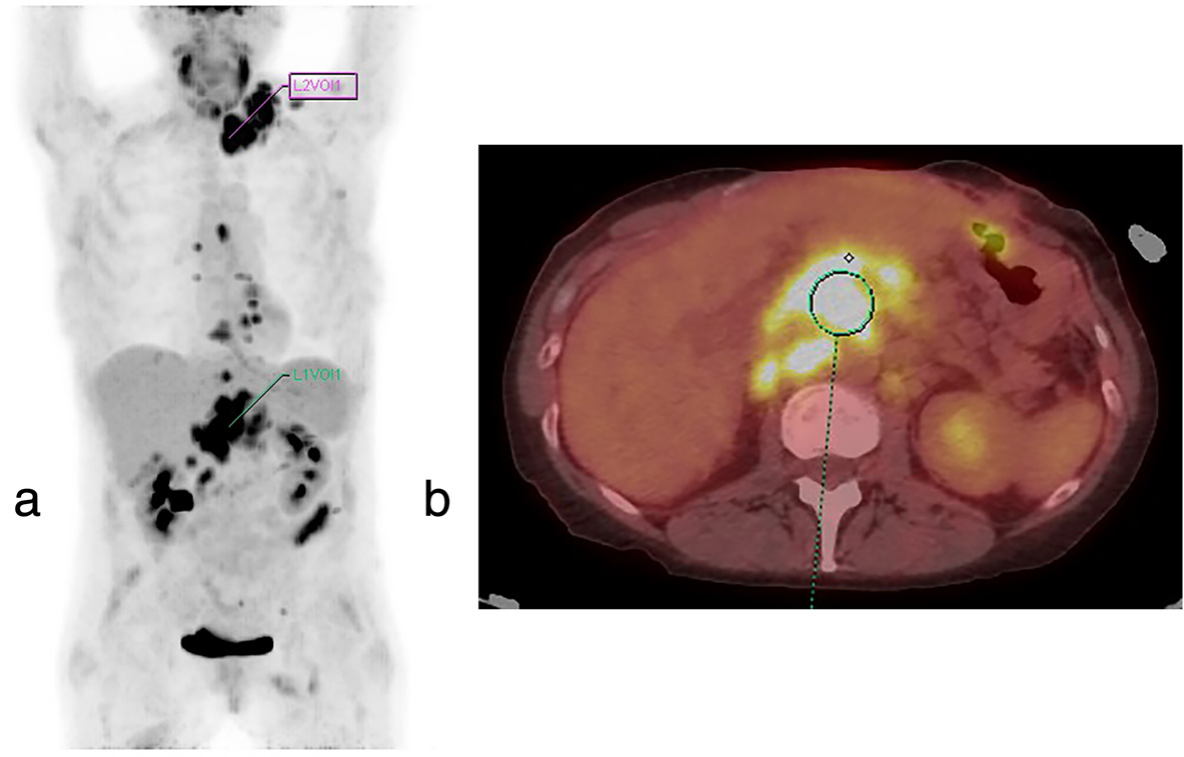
PET/CT of a 52-year-old ovarian cancer patient status post debulking and chemotherapy with active residual disease. Concurrent CA-125 was 89. a PET maximum intensity projection (MIP) image shows intensely FDG avid disease in the left supraclavicular lymph nodes, mediastinal lymph nodes, retroperitoneal lymph nodes, and peritoneal implants. b Axial fused PET/CT image shows cluster of FDG avid retroperitoneal lymph nodes. Volume of interest shows SUV_max_ of 8.67
